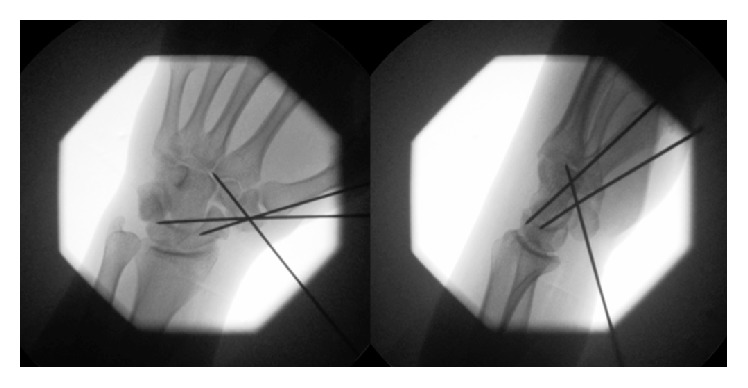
Intraoperative radiographs.
